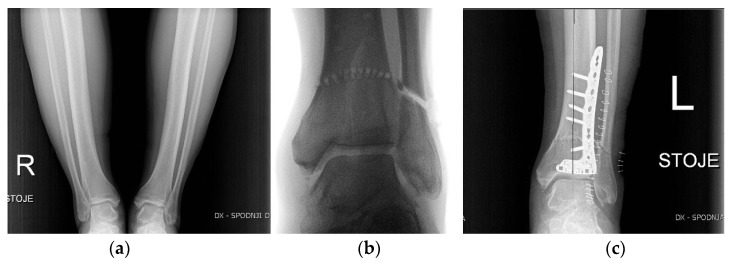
Dome-shaped supra-malleolar osteotomy. (a) preoperative X-ray; (b) intraoperative X-ray; (c) postoperative X-ray.
